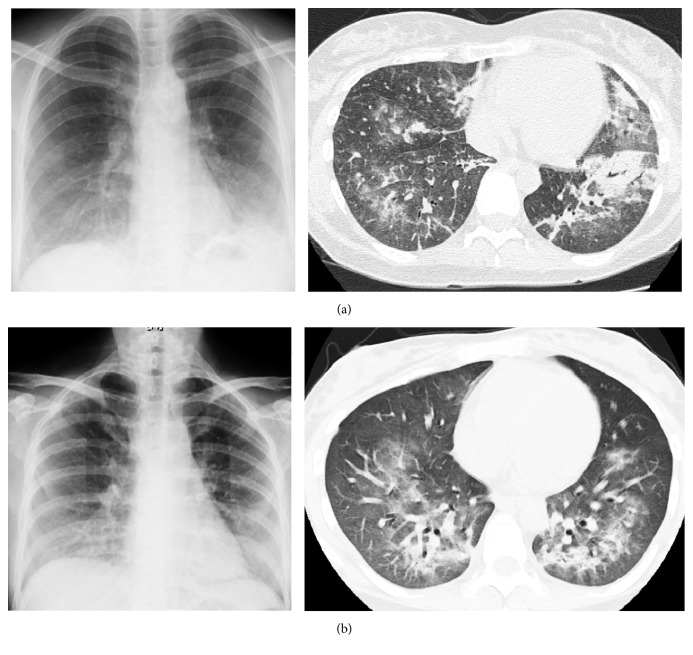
Chest X-ray (left) and computed tomography (CT) (right) images of case  1 (a) and case  2 (b). Chest X-ray showed a high density area in the lower lobes, which was detected as a diffuse ground-glass pattern on CT in both cases.
